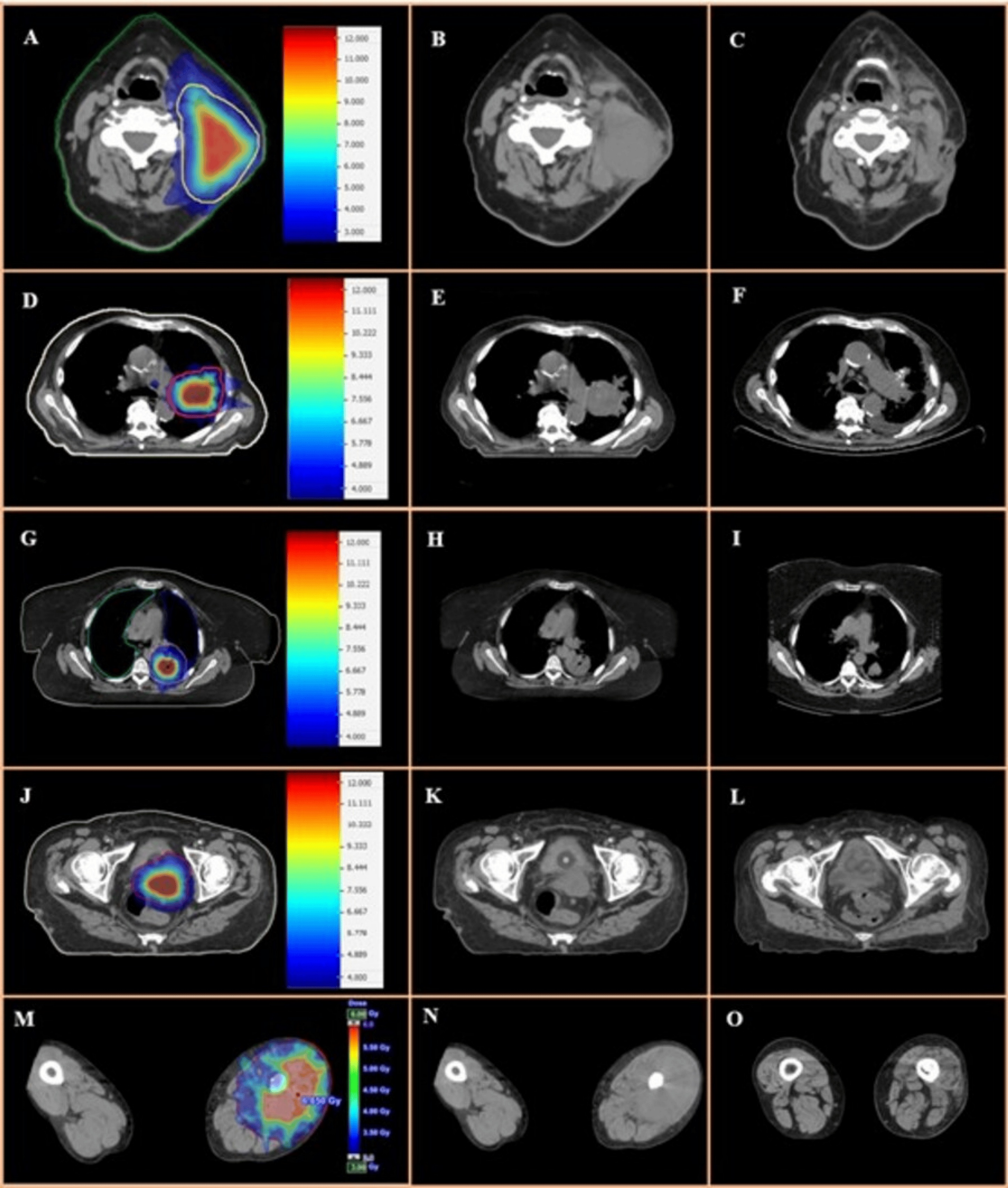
Examples of dose distributions for 2SG-RT and responses observed post treatment (2SG-RT and conventional EBRT). A-C: left cervical lymph-node metastasis from squamous carcinoma (cervical occult primary); A: dosimetry for 2SG-RT, B: diagnostic CT scan, C: CT scan at 2 months post-treatment. D-F: left lung primary adenocarcinoma (hilar lesion); D: dosimetry for 2SG-RT, E: diagnostic CT scan, F: CT scan at two months post-treatment. G-I: left lower lobe (lung) primary squamous carcinoma; G: dosimetry for 2SG-RT, H: diagnostic CT scan, I: CT scan at two months post-treatment. J-L: primary uterine sarcoma; J: dosimetry for 2SG-RT, K: diagnostic CT scan, L: CT scan at two months post-treatment. M-O: right lower limb metastasis from lung adenocarcinoma; M: dosimetry for 2SG-RT, N: diagnostic CT scan, O: CT scan at two months post-treatment.
